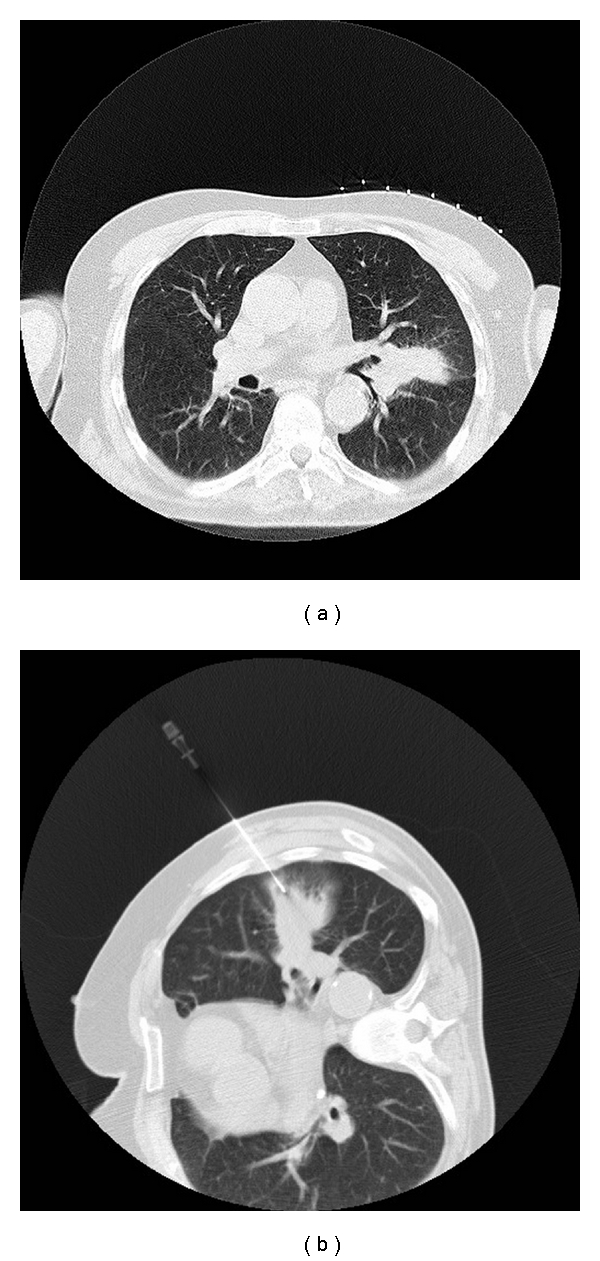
64-year-old man with lung lesion: (a) CT scan of chest in supine position shows left-sided lung lesion. (b) A 19-gauge needle has been positioned within the lesion under CT guidance and core biopsy is then performed using coaxial technique.
